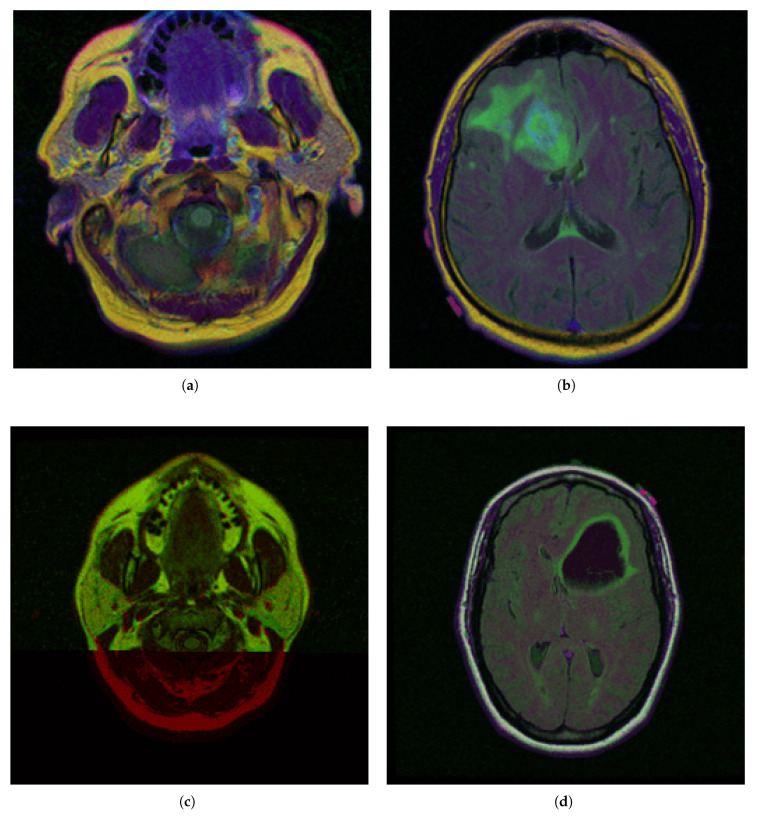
Selected medical MRI dataset from The Cancer Imaging Archive (TCIA) [62] and certified by Duke University experts [63]: (a) TCGA_CS_4941_19960909_3 (CS1), (b) TCGA_CS_4941_19960909_14 (CS2), (c) TCGA_DU_5872_19950223_1 (DU1), (d) TCGA_DU_5872_19950223_35 (DU2).
